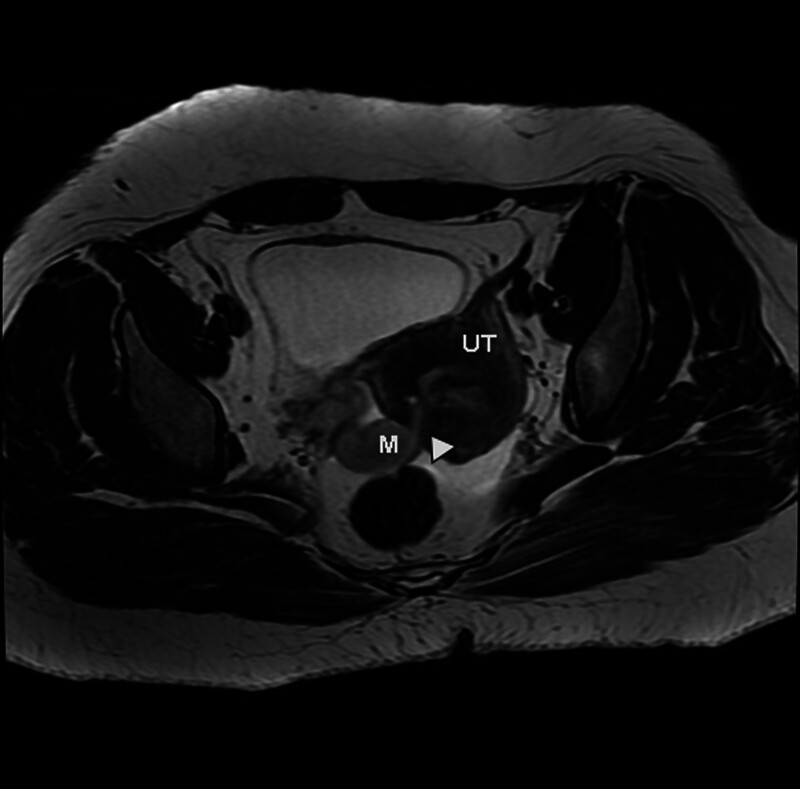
Magnetic resonance imaging (MRI) on axial section exhibited a right parametrial mass of adnexal origin with myometrial invasion (arrow indicates herniated segment of the ipsilateral fallopian tube within the uterine musculature). UT = uterus, M = mass.
